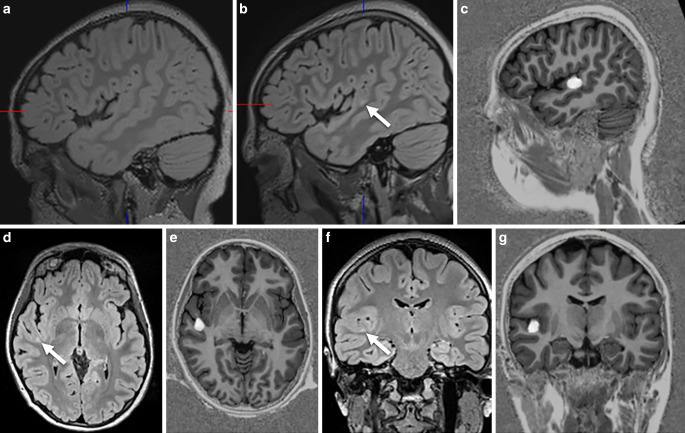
A 14-year-old girl presented with sleep-related, hyperkinetic, tonic and clonic seizures associated with fear since the age of 5. MRI at the age of 5 did not disclose a lesion (a). MRI at the age of 13 showed a slight FLAIR hyperintensity at the gray-white matter border (b,d,f: *arrow*) highlighted by post-processing of MP2RAGE images using the MAP tool (e,g)
